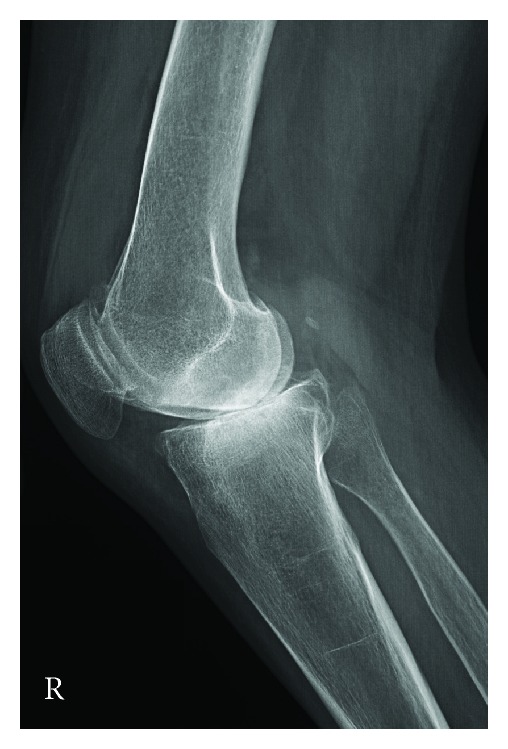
Lateral radiograph of the right knee. A small effusion is present as well.
